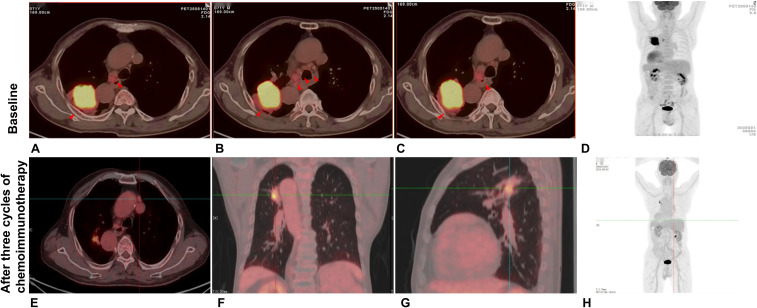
PET-CT comparison before and after neoadjuvant therapy. Initial PET-CT (A–D) reveals a 6.6 cm × 5.2 cm right upper lobe mass (SUVmax 13.7) with hypermetabolic hilar and mediastinal lymph nodes. Post-treatment imaging (E–H) demonstrates significant reduction of the primary lesion to 2.1 cm × 1.7 cm (SUVmax 8.9) and decreased size and metabolic activity of the nodal stations.
